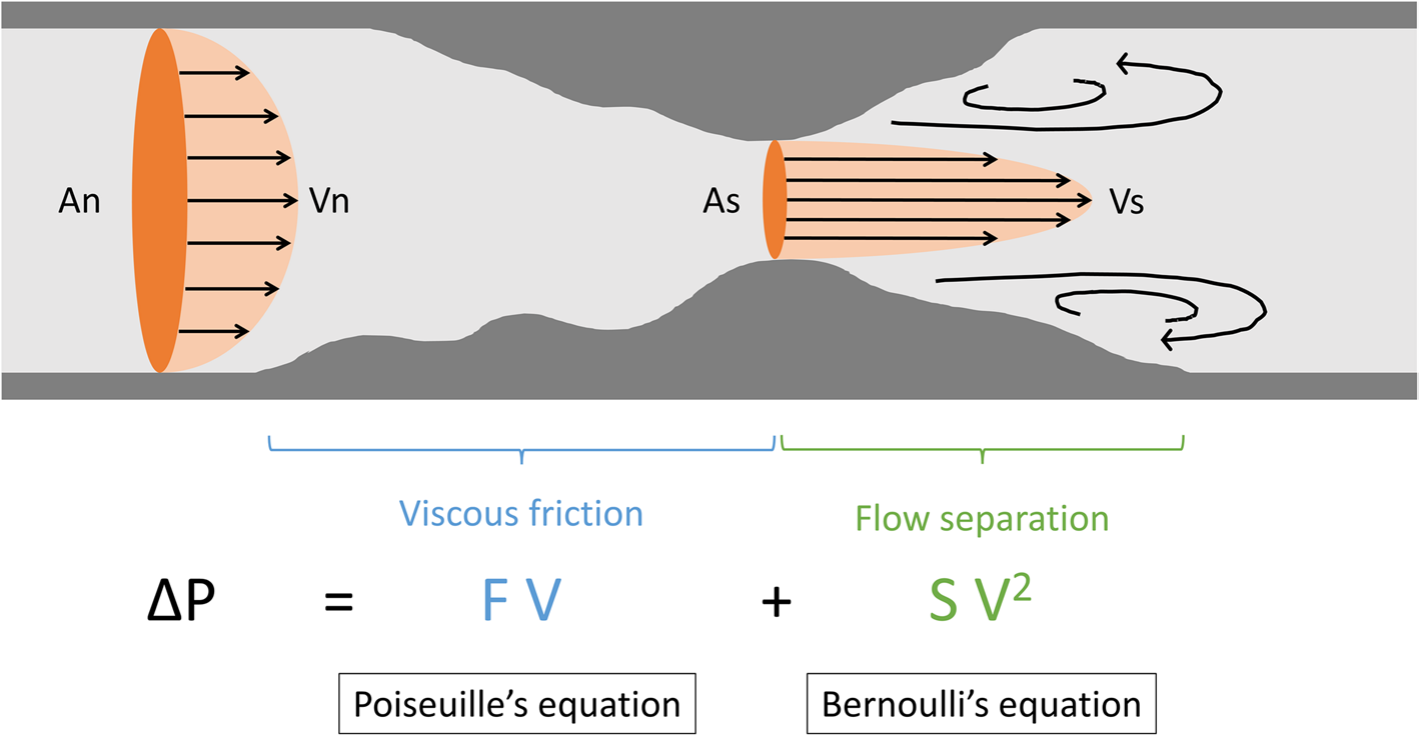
Outline of pressure loss in the stenotic model by fluid dynamics. F is the coefficient of pressure loss because of viscous friction (Poiseuille’s equation), S is the coefficient of local pressure loss because of flow separation (Bernoulli’s equation), and V is flow velocity. F and S were calculated using the following equations: $$\:F=\frac{8\pi\:\mu\:L}{As}\frac{An}{As}$$ , $$\:S=\frac{\rho\:}{2}{\left(\frac{An}{As}-1\right)}^{2}$$. F is calculated as the sum of each IVUS slice, and S is calculated through the whole lesion. The value of µis absolute blood viscosity, set as 4.0 × 10 −3 . L is stenosis length, equal to each slice thickness of IVUS images. An is the cross-sectional area of a normal artery (reference lumen area), As is the cross-sectional area of the stenosis segment, and ρ is blood density(1,050 kg/m 3 ). Abbreviations: An, cross-sectional lumen area of the normal segment; As, cross-sectional lumen area of the stenosis segment; Vn, flow velocity at the normal segment; Vs, flow velocity at the stenosis segment; V, flow velocity; ∆P, pressure loss; L, lesion length; IVUS, intravascular ultrasound.
